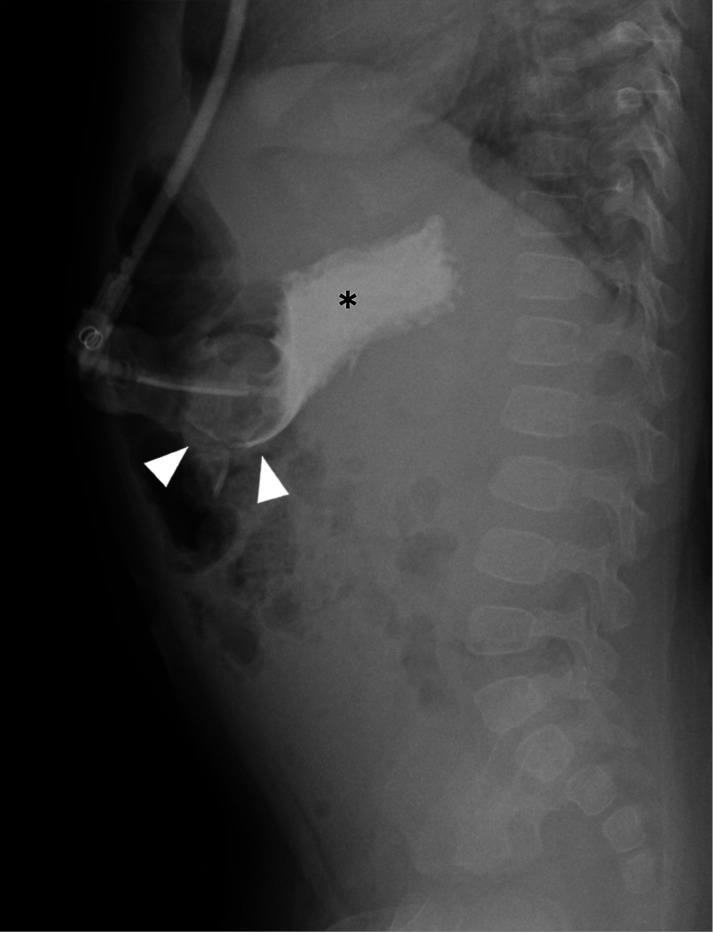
Cross-table lateral abdomen radiograph shows intraluminal contrast flow into the gastric lumen (*) and gastric contrast outlining the tube retention balloon (arrowheads).
